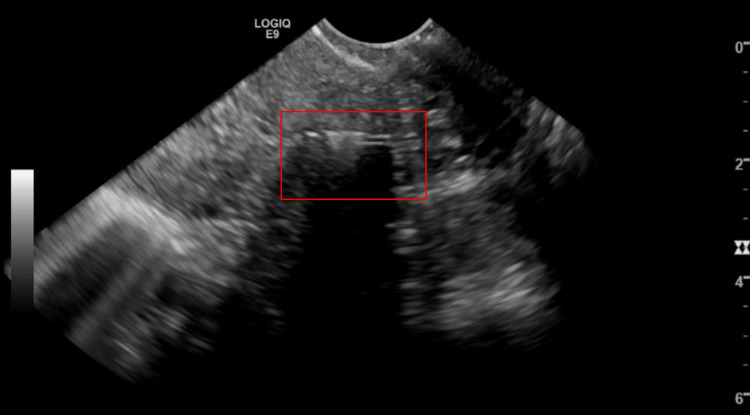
Intrauterine device fragment redemonstrated on endovaginal ultrasound in sagittal view, with a red box highlighting retained fragment.
